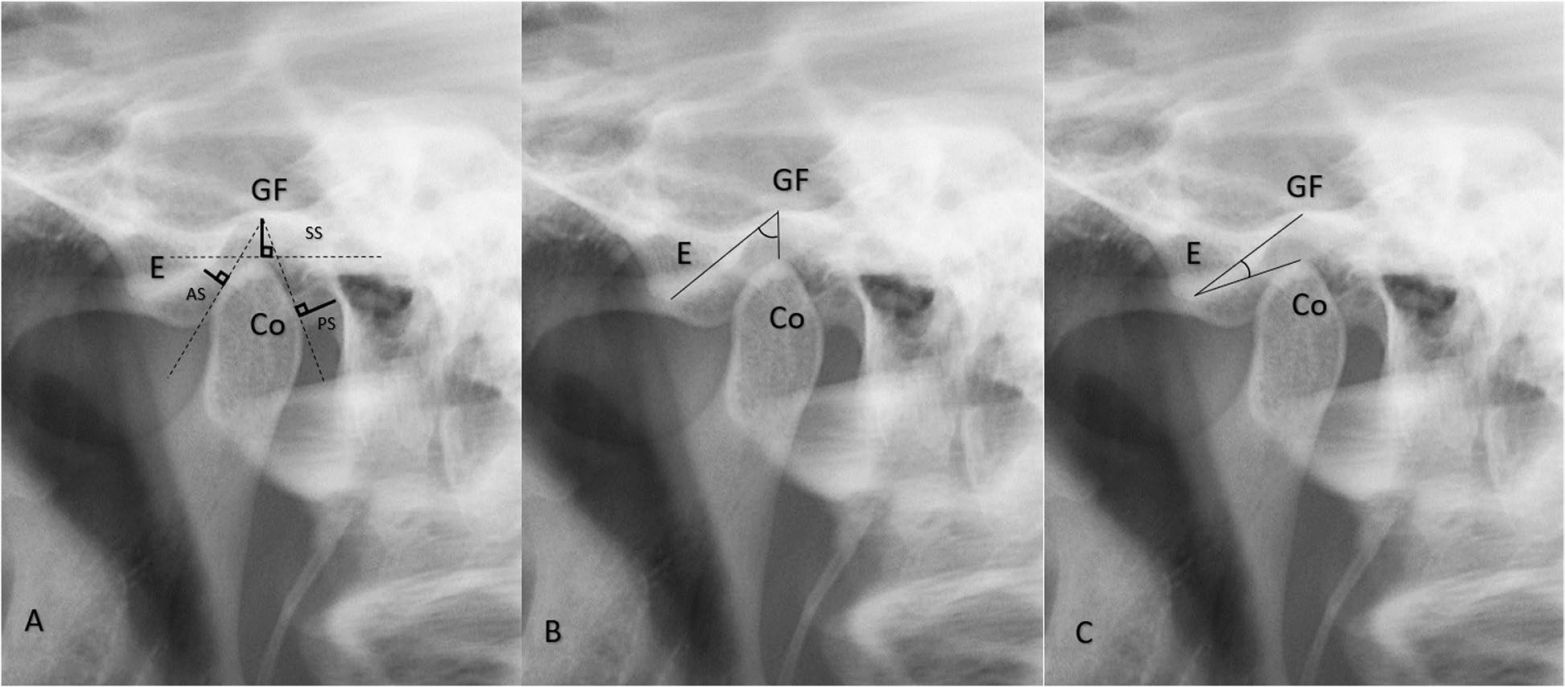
TMJ measurements in OPT. A Distance measurements B. Antero-posterior Angle (APA) C. Supero-inferior Angle (SIA). OPT: Orthopantomographic Co: Condyle, E: Articular eminence, GF: Glenoid Fossa, AS: Anterior Space, SS: Superior Space, PS: Posterior Space
